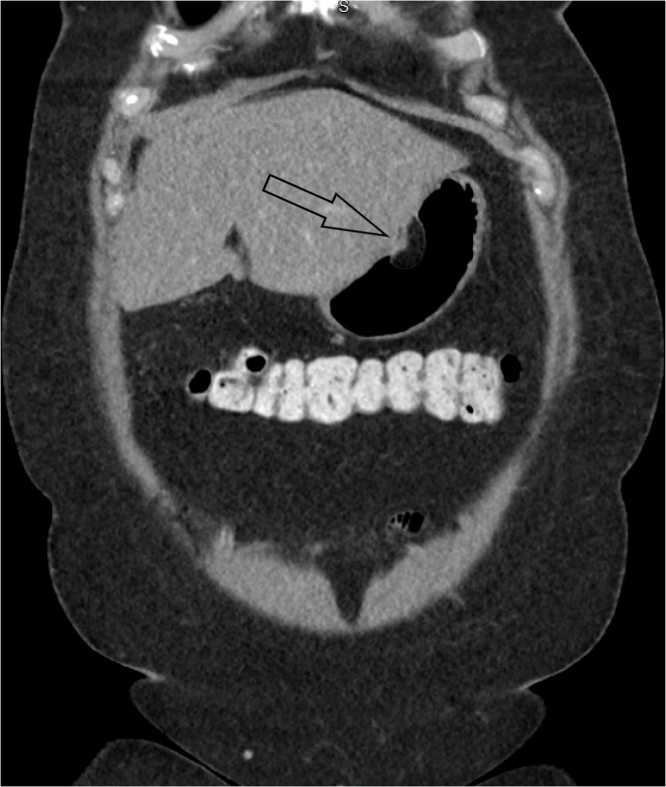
coronal post-contrast CT images show a well-circumscribed, smoothly marginated, ovoid intramural (submucosal) mass of uniform fat attenuation, arising from the lesser curvature of the body of the stomach with endoluminal extension. The mass measures 1.5 × 1.5 × 3.0.
